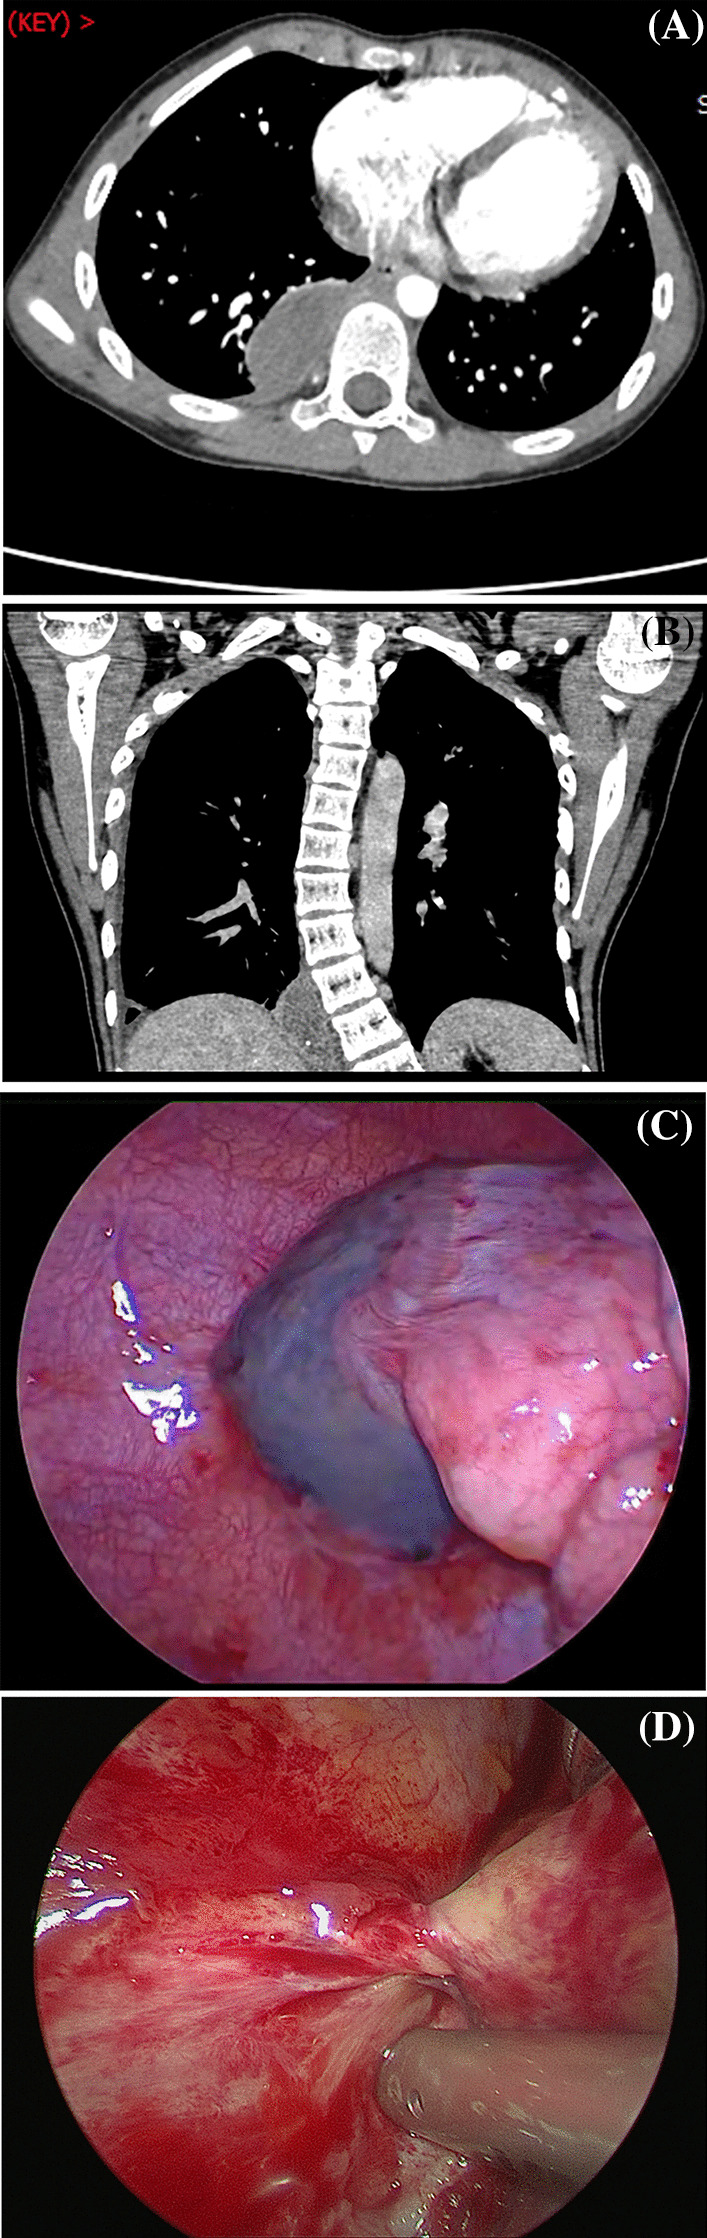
Axial Contrast-enhanced CT image of the chest A indicates that a well-bordered, soft-tissue density mass without enhancement in the right thoracic cavity, coronal CT image B shows a well-defined soft tissue mass between the diaphragm and the spine, video-assisted thoracoscopy C reveals a purplish-red mass with congestion and necrosis in the pleural cavity, and D shows a twisted vascular pedicle
